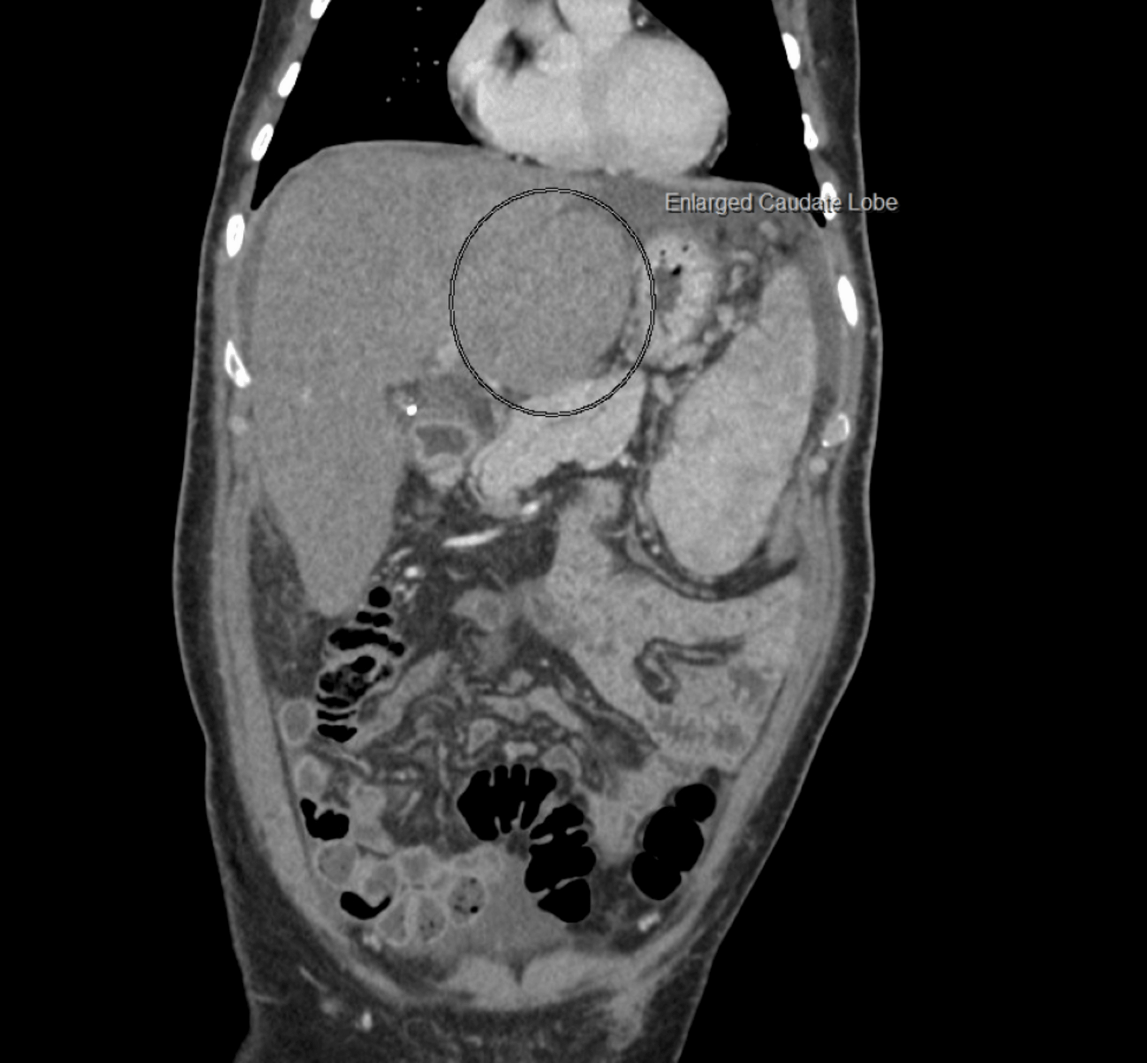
Abdominal CT image showed an enlarged caudate lobe (as indicated within the circle)
